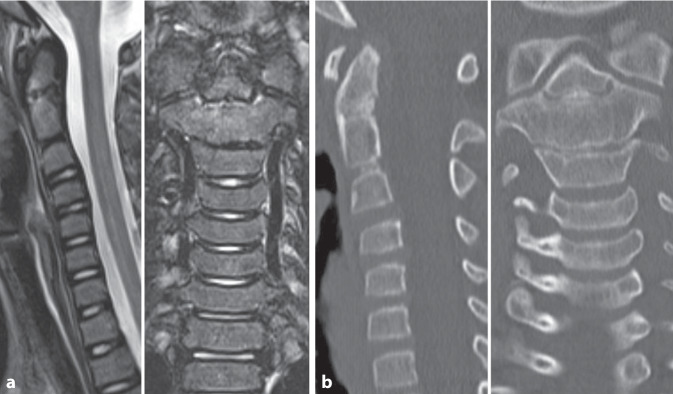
In der Verlaufs-MRT nach 3 Monaten (a) zeigt sich weiterhin ein ventral betontes Ödem an der Densbasis, woraufhin eine CT indiziert wurde (b). Hier findet sich eine progrediente Verknöcherung, ohne Anhalt auf Dislokation (b)
